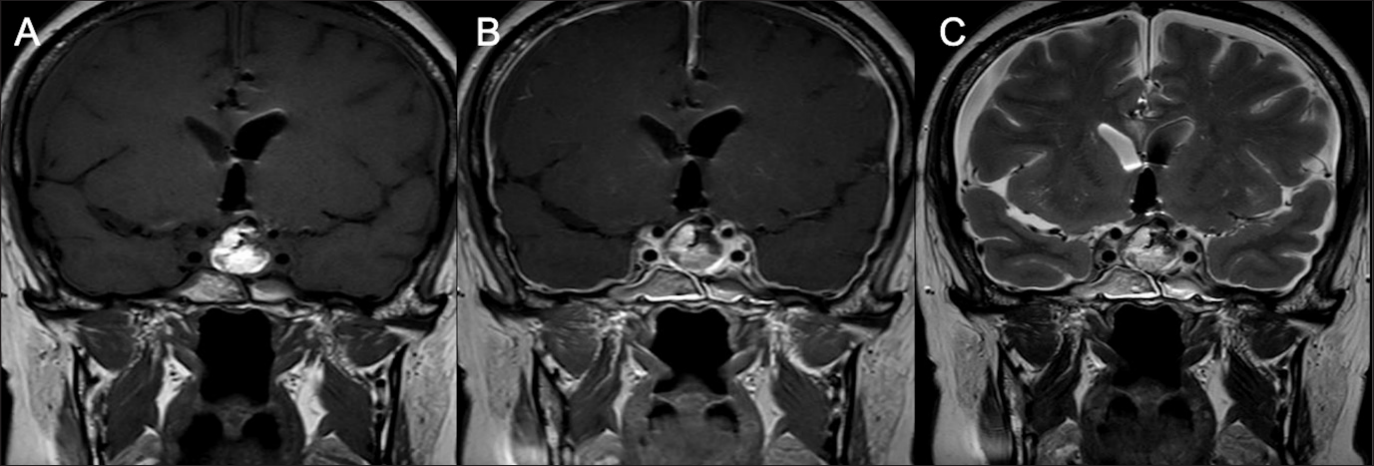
MRI after first surgery: Coronal T1WI (A), Coronal post-contrast T1WI (B) and coronal T2WI revealed signs of intracranial hypotension: right and left subdural collections and pachymeningeal thickening and enhancement.
